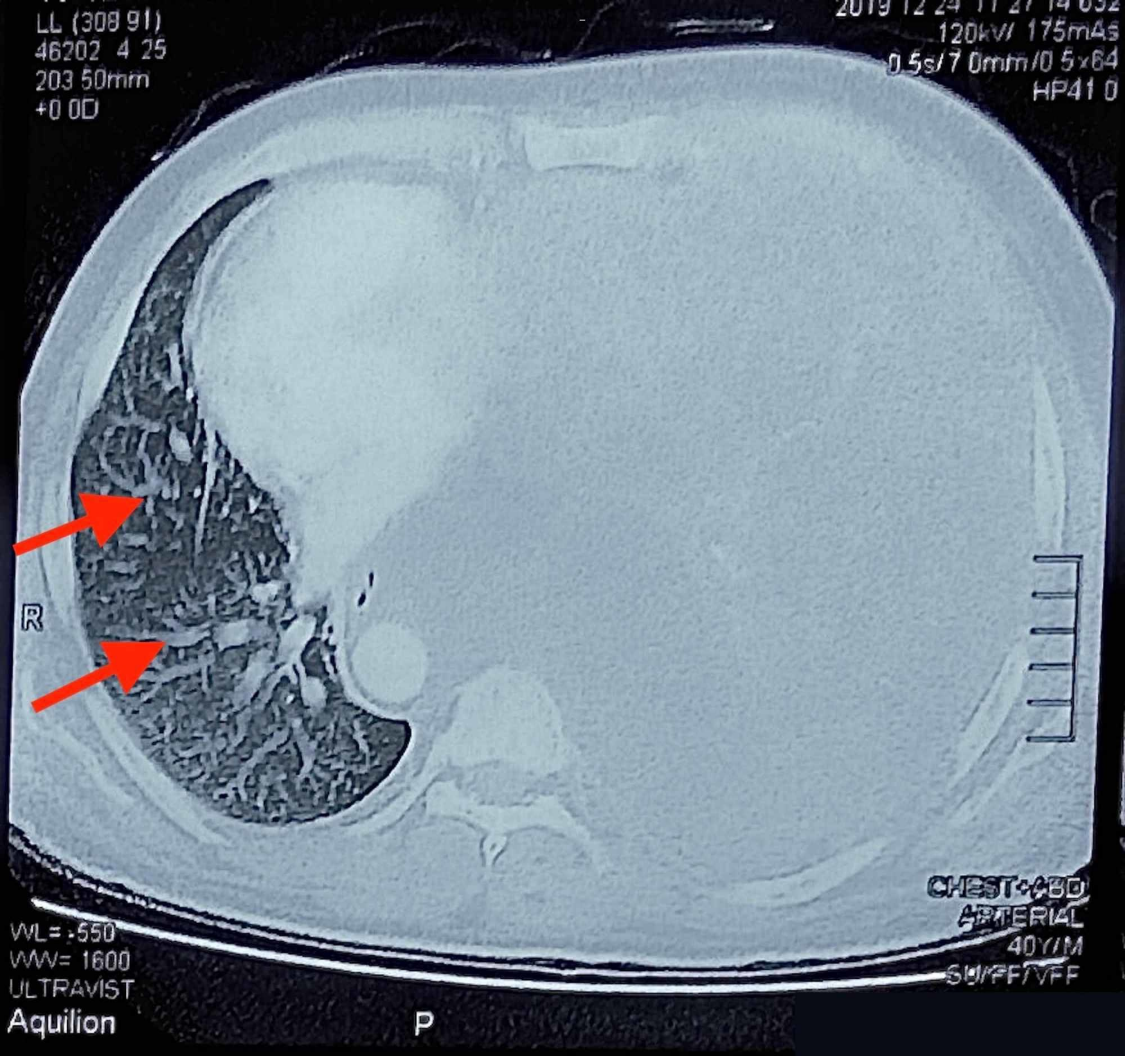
A chest CT showing a large lobulated circumferential mass filling the left hemithorax with multifocal extensions through diaphragm, chest wall and mediastinal fats. Enlarged lymph nodes in the left hilar, tracheobronchial and subcarinal regions are noted. Ground-glass opacities are also seen (red arrows). CT: computed tomography.
